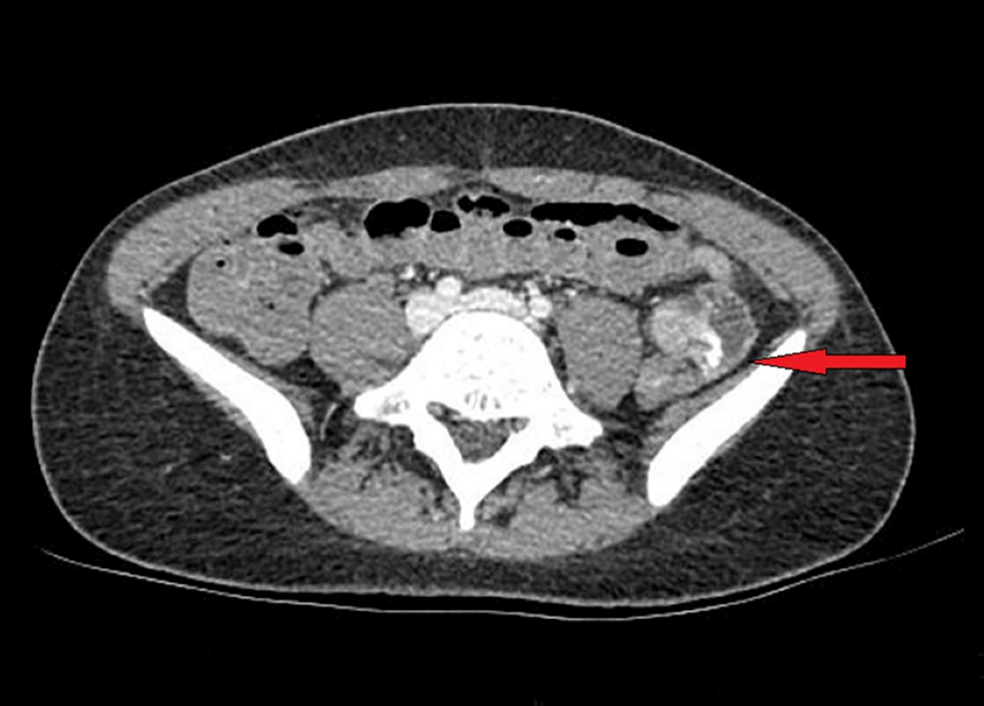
Abdominal CT angiography depicting contrast extravasation in the distant ileum (indicated by red arrow)
